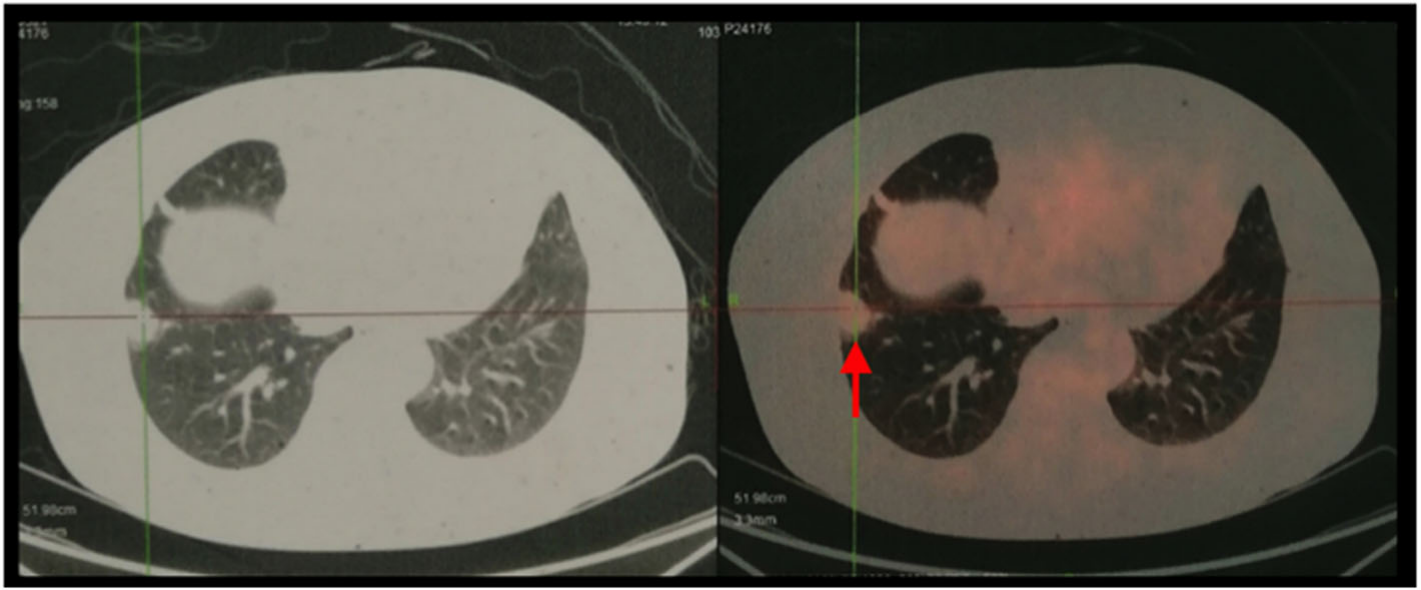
PET-CT showed mild uptake of 18F-FDG (SUVmax 2.1, arrow)
